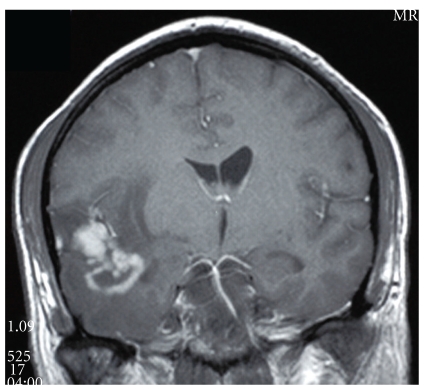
Central nervous system Hodgkin's lymphoma. Computed tomography image demonstrating an ill-defined ring-enhancing intracranial lesion with peritumoural edema.
